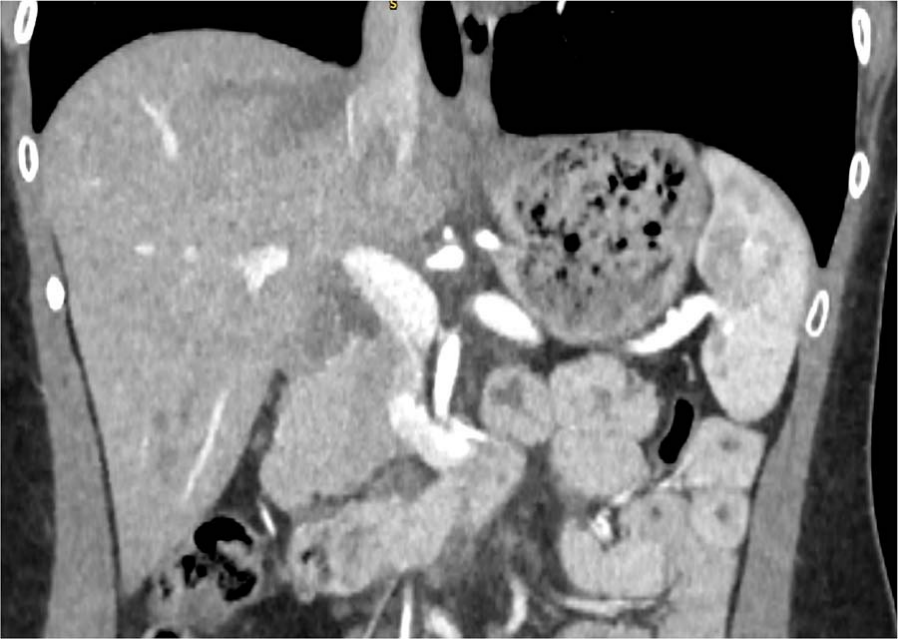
CT pancreas demonstrating absent body and tail of pancreas in coronal view.
